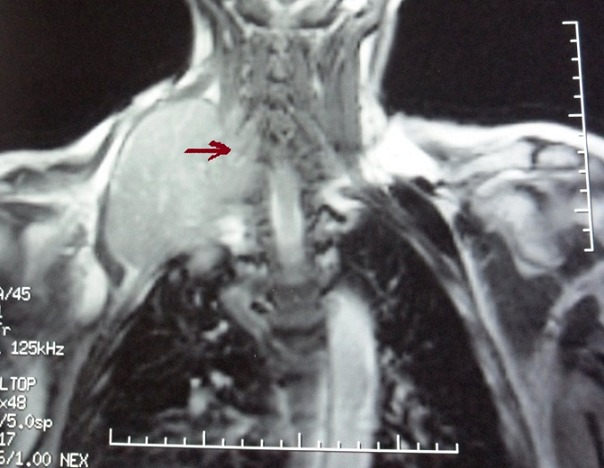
Spinal MRI, frontal view: a large right supraclavicular mass with dorsal extension at C7-D1
